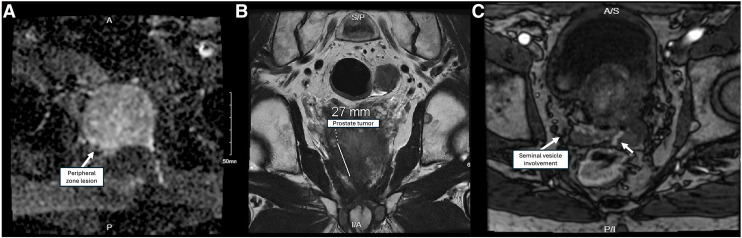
Progression of primary prostate tumor seen on MRI. (A) Subcentimeter lesion in right posteromedial peripheral zone seen in 2014 and 2016. Two other subcentimeter lesions not seen here are present in right posteromedial peripheral zone. (B) By 2024, this lesion grew to 2.7 cm and expanded from base to apex, crossing midline to left. (C) Bilateral lesions that involve right seminal vesicle and potentially left seminal vesicle.
